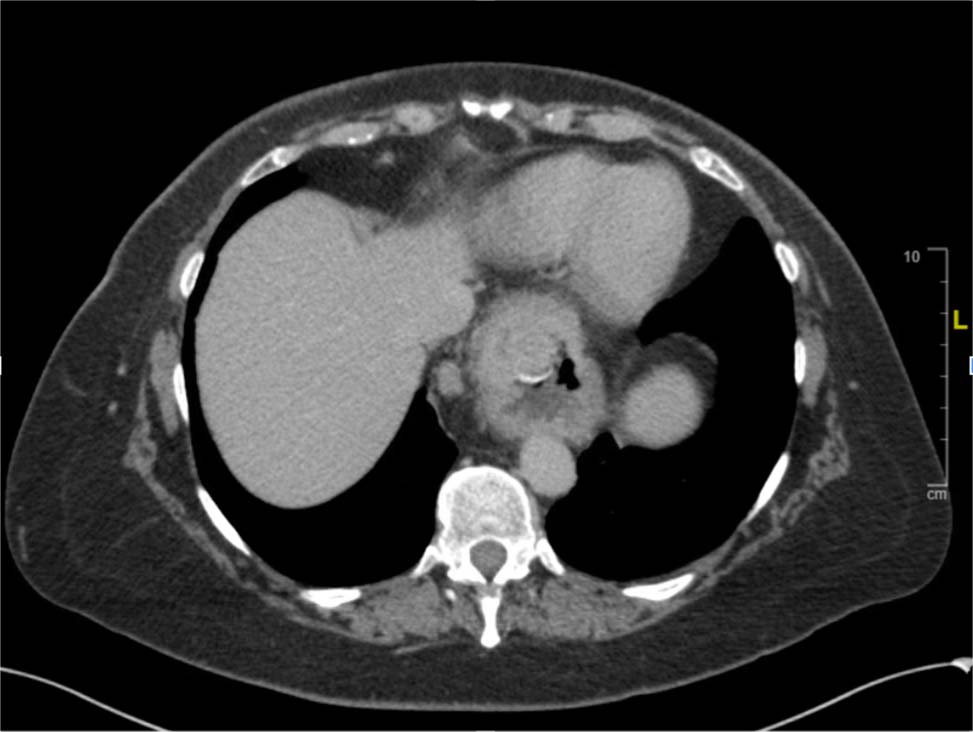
Computed tomography imaging with contrast demonstrating an intraluminal soft tissue lesion within the hiatal hernia measuring approximately 2.3 cm in transaxial dimension. There is an adjacent borderline enlarged paraesophageal lymph node measuring 9 mm.
